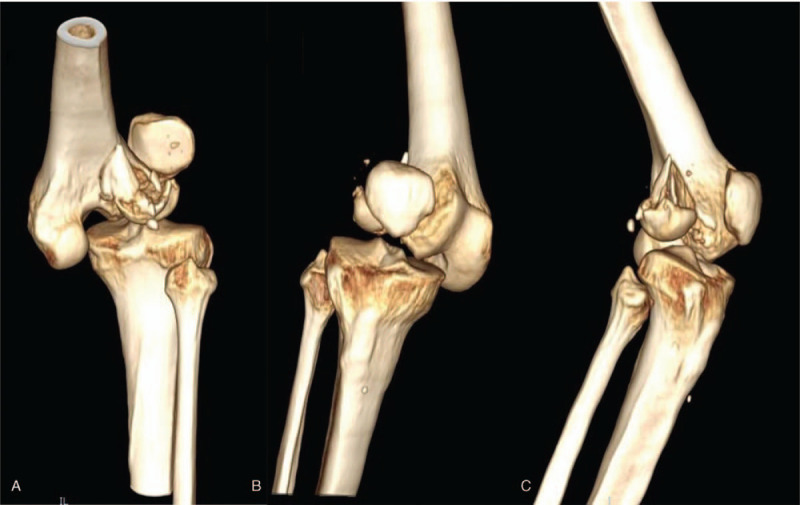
(A–C) The CT of knee joint showed the comminuted lateral Hoffa fracture combined with rotational dislocation of the knee joint.
